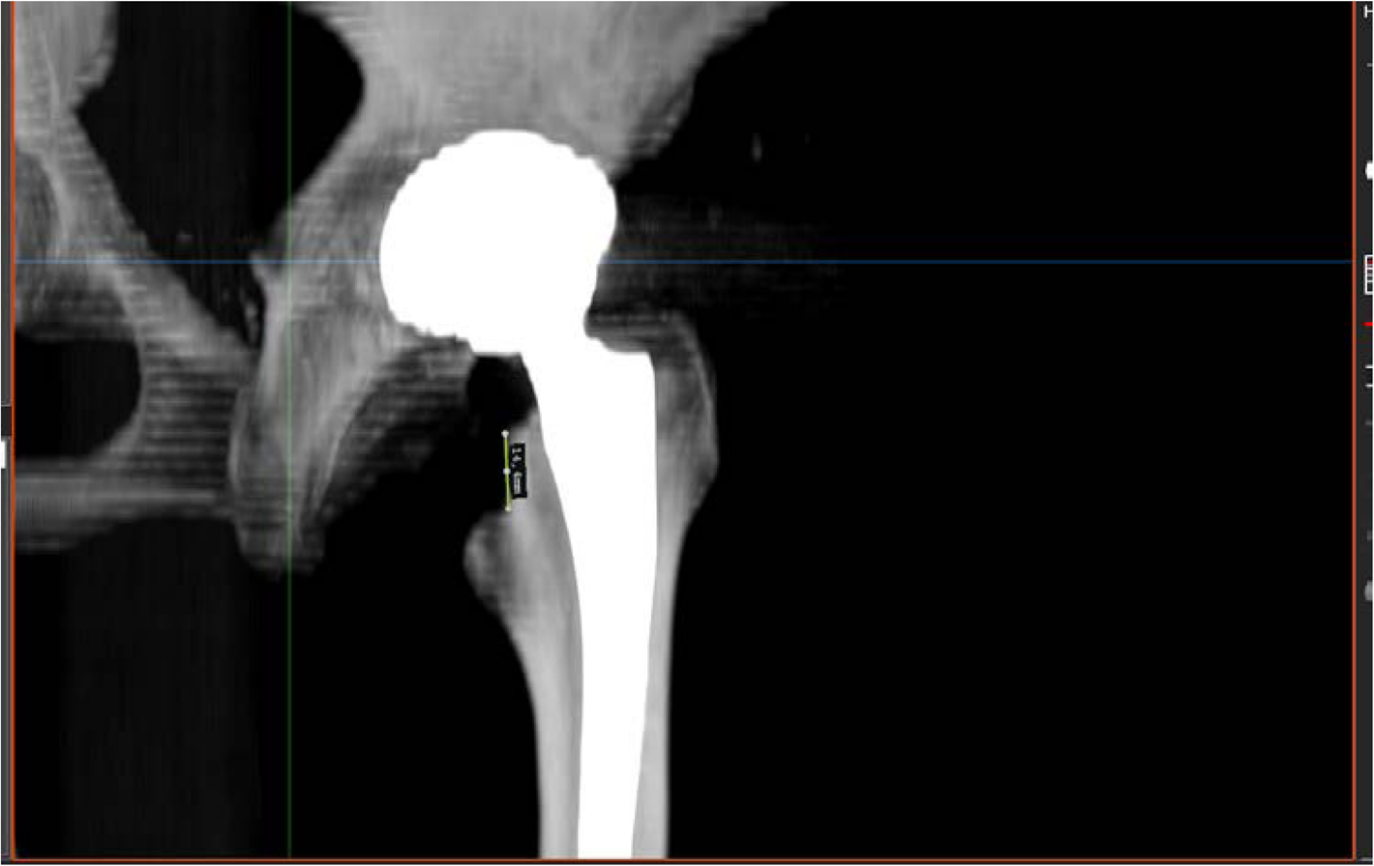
Measurement of the femoral neck resection height by using the ‘semi-automatical’ function of a newly developed digital planning software for CT scans (Modicas, Erlangen, Germany)
